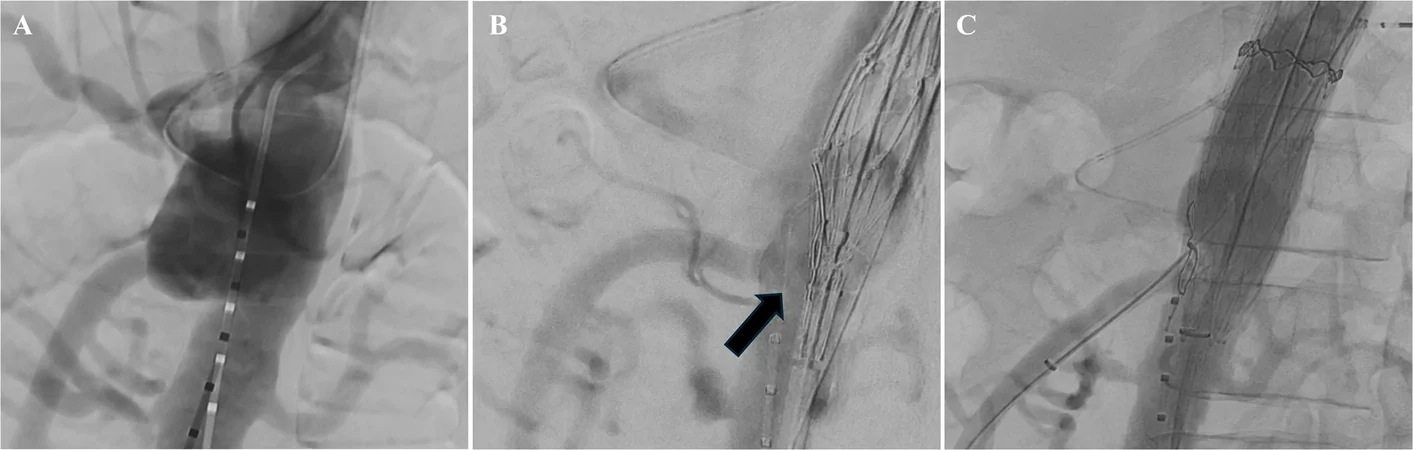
Antegrade approach (Case 3). A Preoperative imaging of a Crawford type V thoracoabdominal aortic aneurysm. The distance from the distal aneurysm margin to the left and right renal arteries was 15 mm and 20 mm, respectively. The superior mesenteric artery (SMA) was designated as the target vessel for fenestration, necessitating concomitant celiac artery embolization. Adequate collateral perfusion via the gastroduodenal artery (GDA)—initially evaluated on preoperative computed tomography—was intraoperatively verified by SMA angiography during temporary balloon occlusion of the celiac artery. An antegrade access strategy was selected because of the acute distal angulation of the SMA, following confirmation of minimal thoracic aortic mural thrombus. B Following optimal positioning, the front stop is released, and the release sleeve is opened to fully deploy the proximal segment. Maintaining distal constraint (back stop) secures a critical working space between the fenestrated endograft, the aortic wall, and the target branch, thereby facilitating antegrade cannulation (arrow). C Analogous to the retrograde approach, a 100-cm, 7-Fr guiding sheath (Parent Plus; Medikit) is advanced into the target vessel. The distal segment of the endograft is then fully deployed, followed by the introduction, deployment, and flaring of the bridging stent
